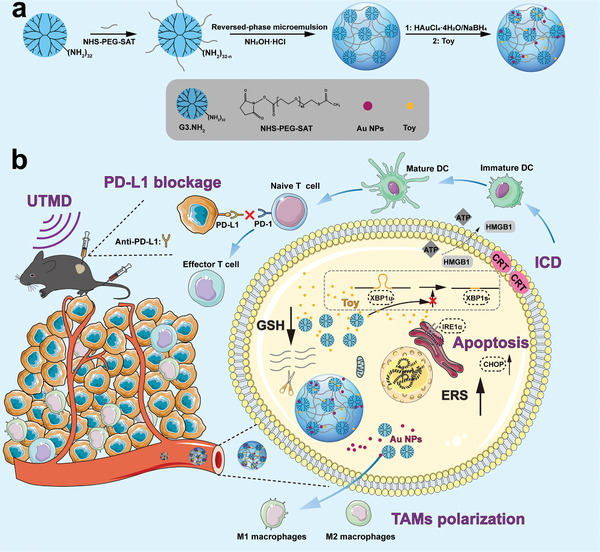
Construction of Au/Toy@G3 NGs (a) for UTMD‐enhanced chemoimmunotherapy and CT imaging of pancreatic tumor in combination with anti‐PD‐L1‐mediated ICB (b).
